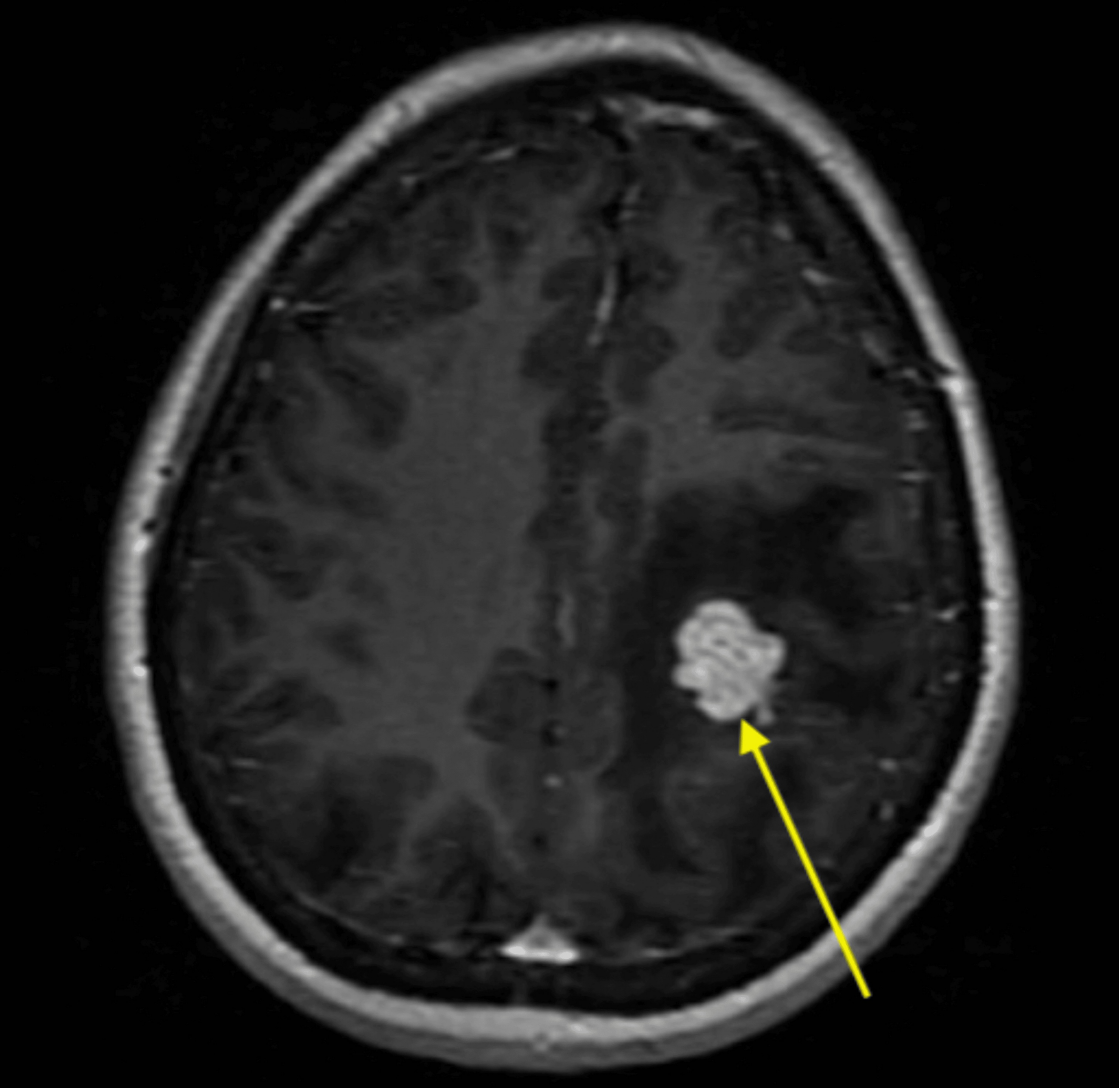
Axial T1-weighted post-contrast MRI showing an enhancing lesion in the left parietal lobe (yellow arrow) with surrounding vasogenic edema.
